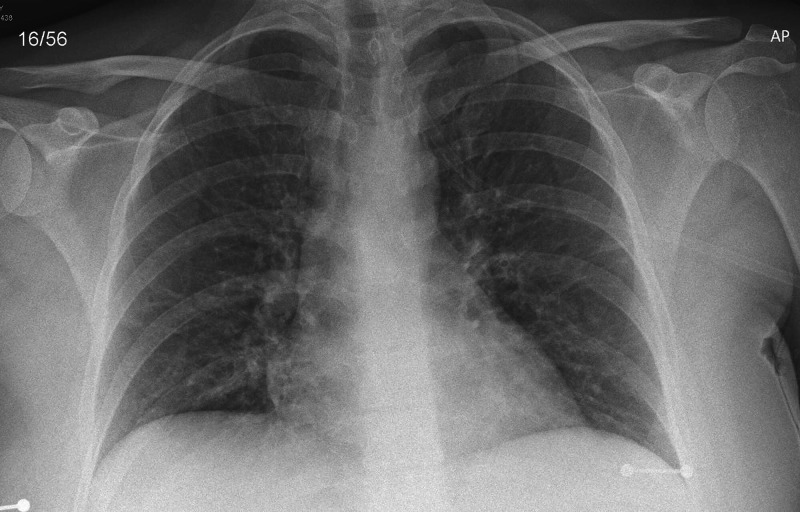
Normal chest X-ray on admission (22/09/2020)
